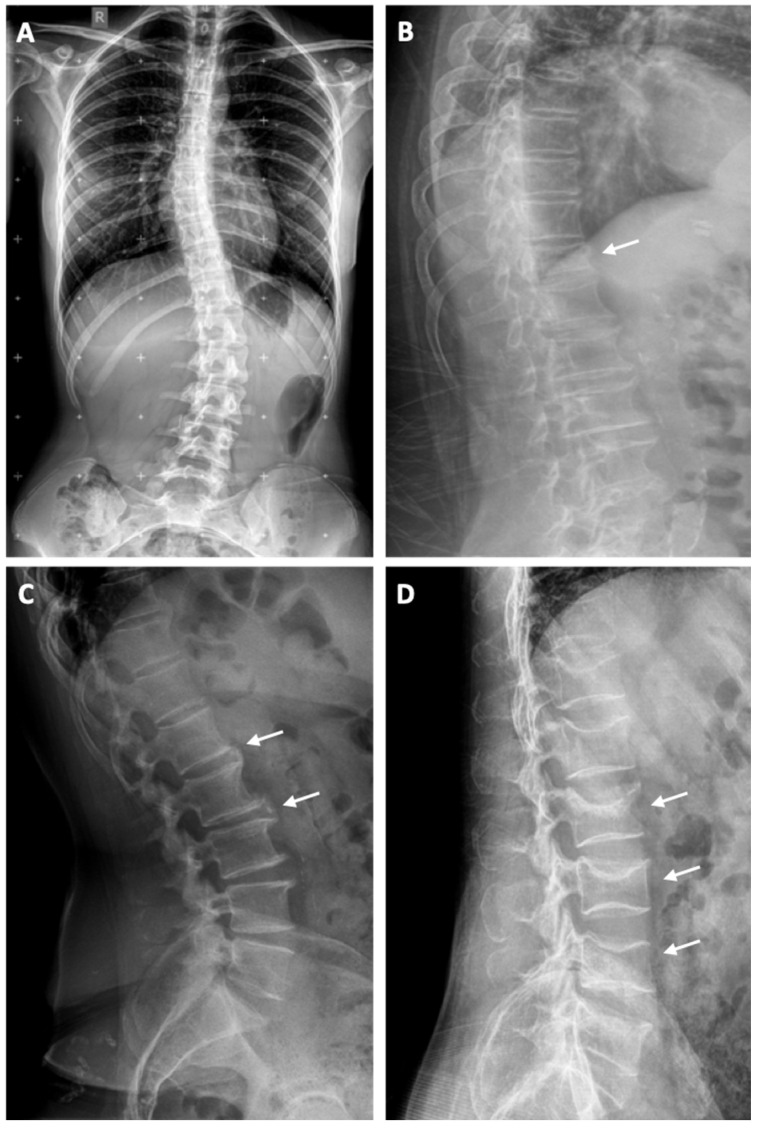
Assessment of the plain radiograph features with spine abnormalities in different patients. (A) Anteroposterior long-cassette radiograph of an adolescent idiopathic scoliosis in a 15-year-old patient with Risser 3; (B) Lateral plain lumbar spine x-ray reveals a typical wedge-shaped vertebral deformity (white arrow), indicating a L1 fracture; (C) Lateral lumbar spine plain x-ray reveals moderate disc space narrowing and anterior osteophytes (white arrows), indicating degeneration of the lumbar disc; (D) Lateral lumbar spine plain x-ray reveals biconcave deformity of their endplates and loss of more than 30% of the vertebral height (white arrows) in a patient with idiopathic osteoporosis.
